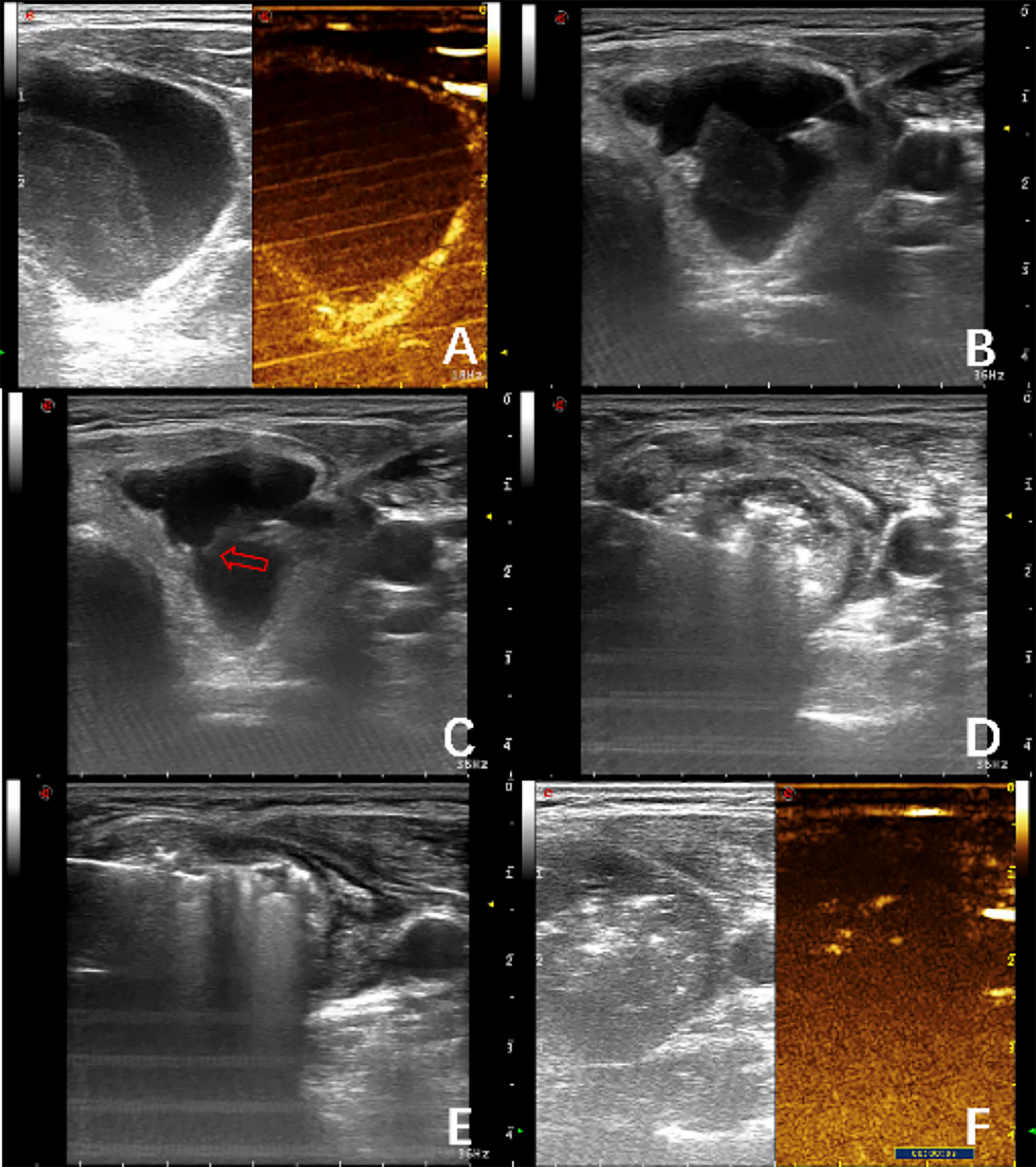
A 50-year-old woman presented with cystic solid nodules in the left lobe of the thyroid gland, about 4.1 cm × 3.7 cm × 2.8 cm in size. 70%, preoperative contrast-enhanced ultrasound showed abundant blood supply in the cyst wall and the internal solid part of the nodules and the contrast-enhanced image showed equal enhancement. (A) Aspiration of cyst fluid. (B) The nodules were bleeding in the cyst, and the bleeding spots on the cyst wall were squirted (arrows). The deflated cyst cavity was filled again, and the cavity was clouded (C). Hematoma formed inside the nodules (D) Mobile layer-by-layer microwave ablation (E) was performed for solid components of nodules and hematoma. Immediate postoperative angiography, no contrast filling in and around the nodules (F).
